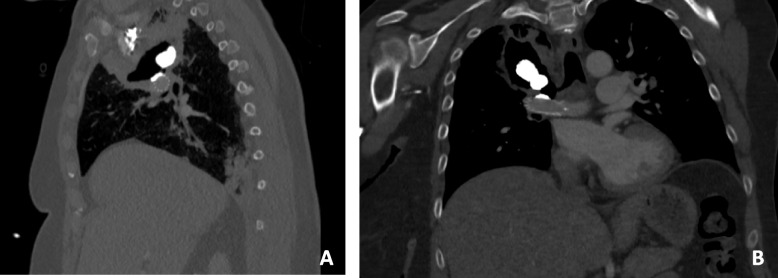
CT scan (day 10) showing a completely excluded pseudoaneurysm and no bleeding around the stent in sagittal A and coronal B section
